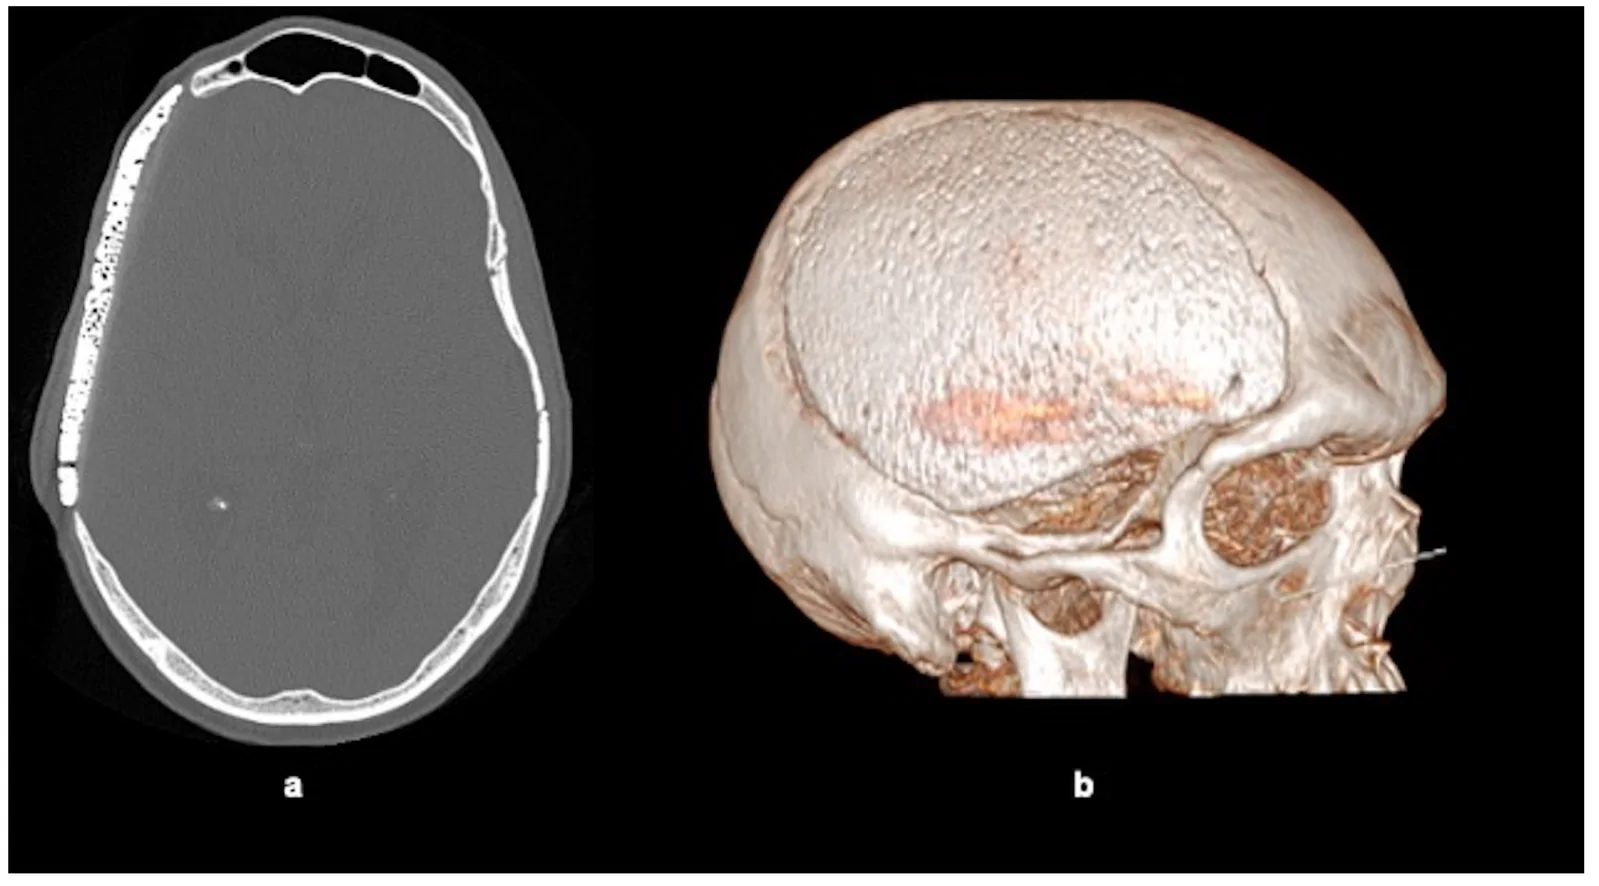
Bone CT-scan 48 h post-operatively: (a) axial bone CT image showing satisfactory placement of the cranioplasty flap; (b) 3D reconstruction of the CT scan.
